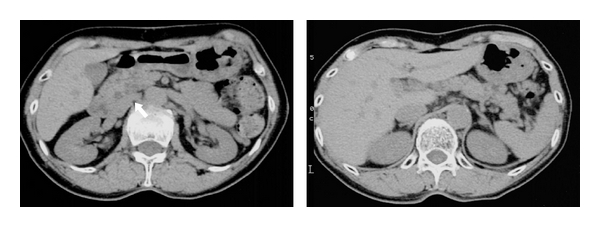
Case 2: Noncontrast CT showing a small multilocular cystic lesion in the head of the pancreas and no lesions in the tail of the pancreas.
